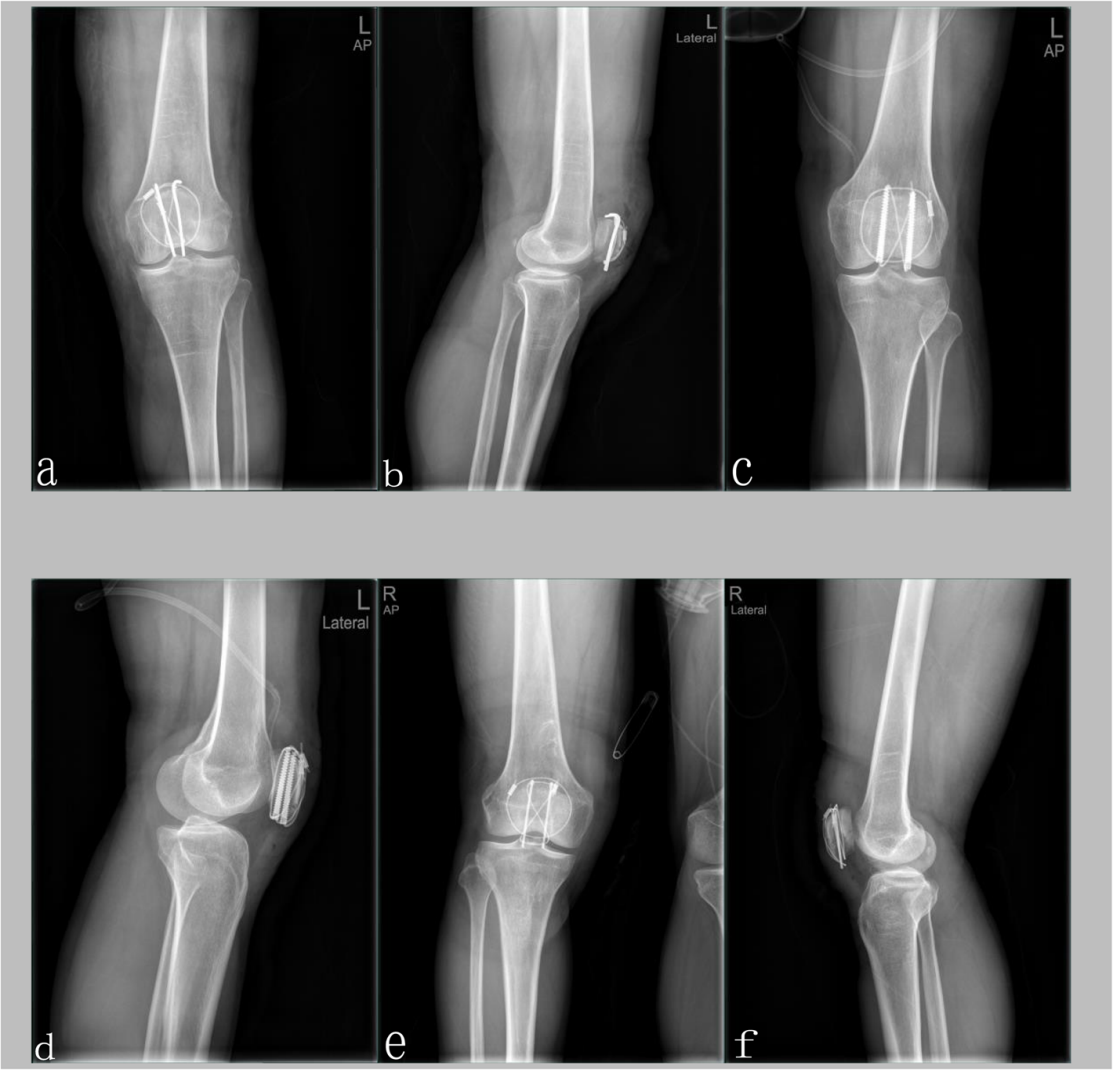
a AP radiograph of the knee, showing a comminuted patellar fracture fixed by MKTB. b On lateral radiograph, the articular step should be less than 1 mm. c AP radiograph of a case one day after undergoing fixation of CSTB. d The Lateral radiograph of the former case. e RPTB was used in this case. The tension bands were constructed in a figure-eight pattern. f The interfragmentary gap must be less than 2 mm
